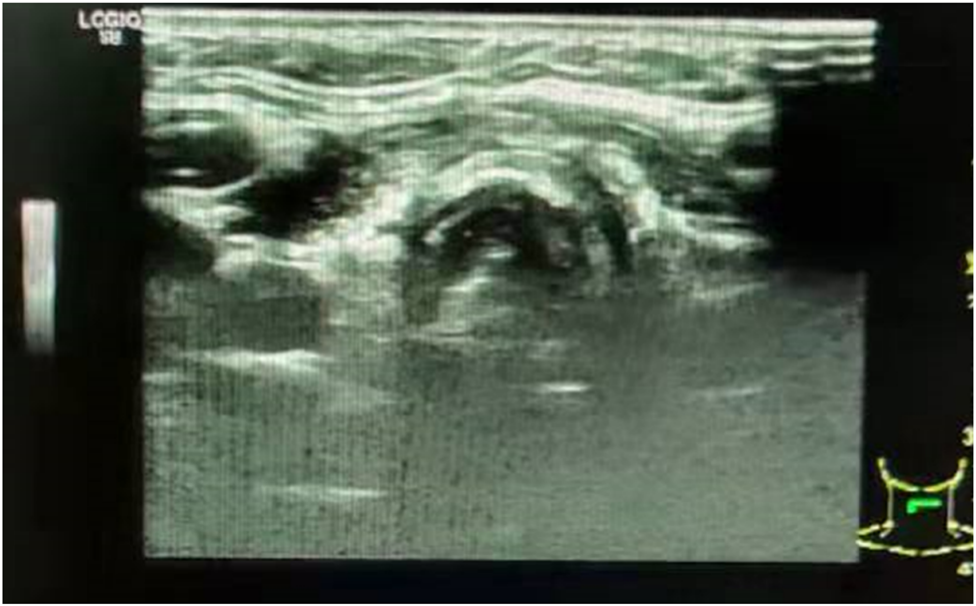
Thyroid color ultrasound: no obvious abnormal echo was observed in the thyroid area.
